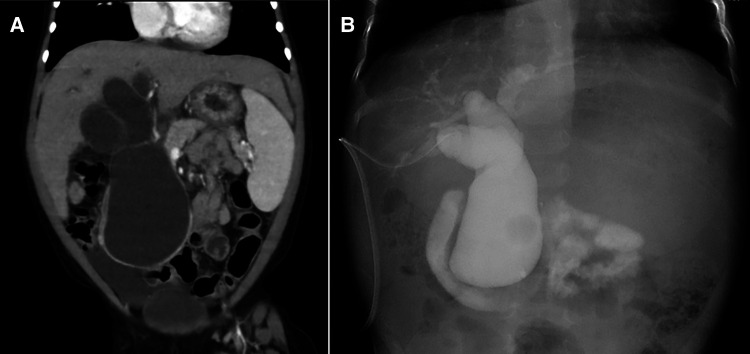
Diagnostic imaging findings (A) contrast-enhanced computed tomography (B) cholangiography.
